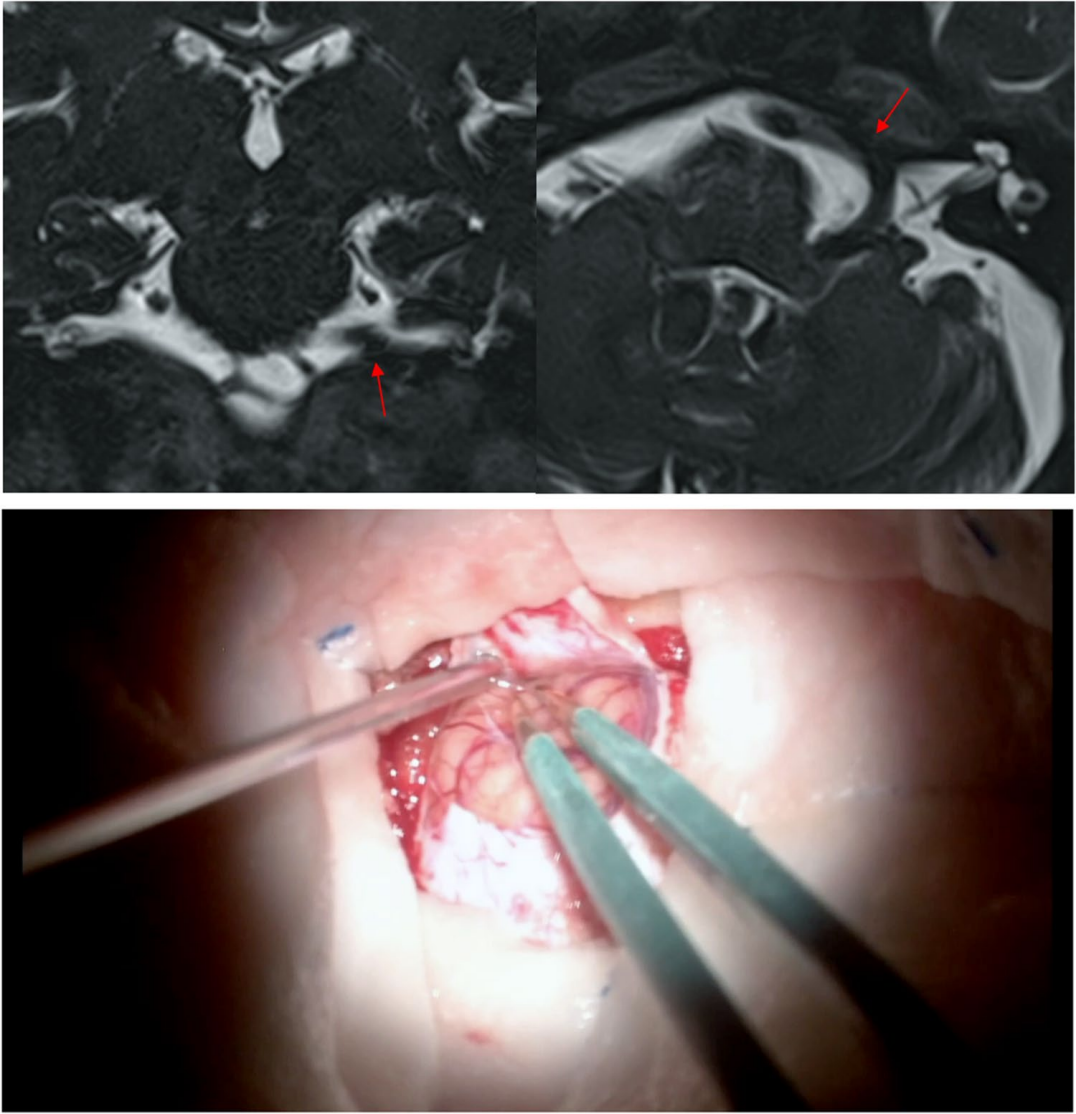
The brain MRI with FIESTA sequences in the coronal and axial planes showed the fibrous attachment between the left VA and the petrous bone dura mater (red arrow)
